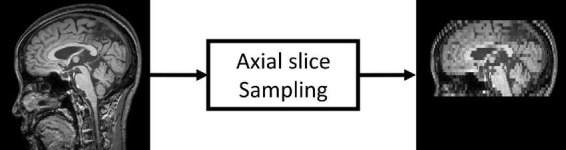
Low-resolution magnetic resonance imaging (LRMRI) image generation. LRMRI images were generated from high-resolution magnetic resonance imaging (HRMRI) images in this study by sampling a maximum total of 25 slices in an axial plane containing cerebral in uniform intervals. After sampling, the slice thickness becomes 0.5–1.25 mm to 4.5–5.5°mm.
